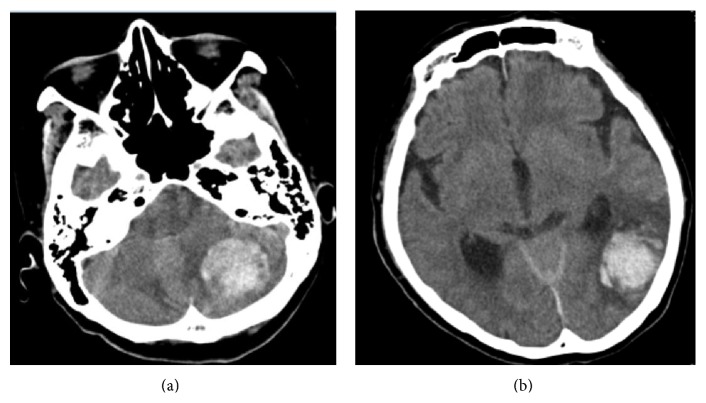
Hemorrhage was observed in both the left cerebellum (a) and the temporal lobe (b) 5 days after spine surgery on CT.
